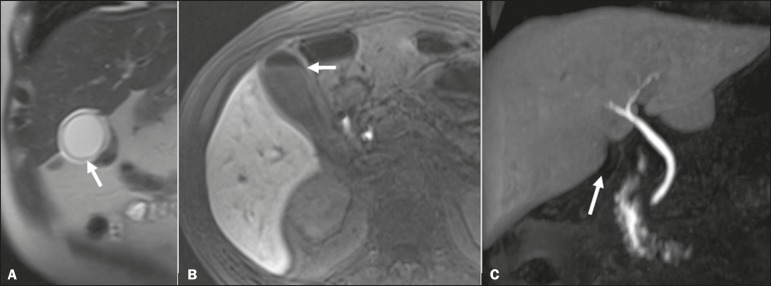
Acute cholecystitis. Fast spin-echo axial T2-weighted MRC sequence (A)
